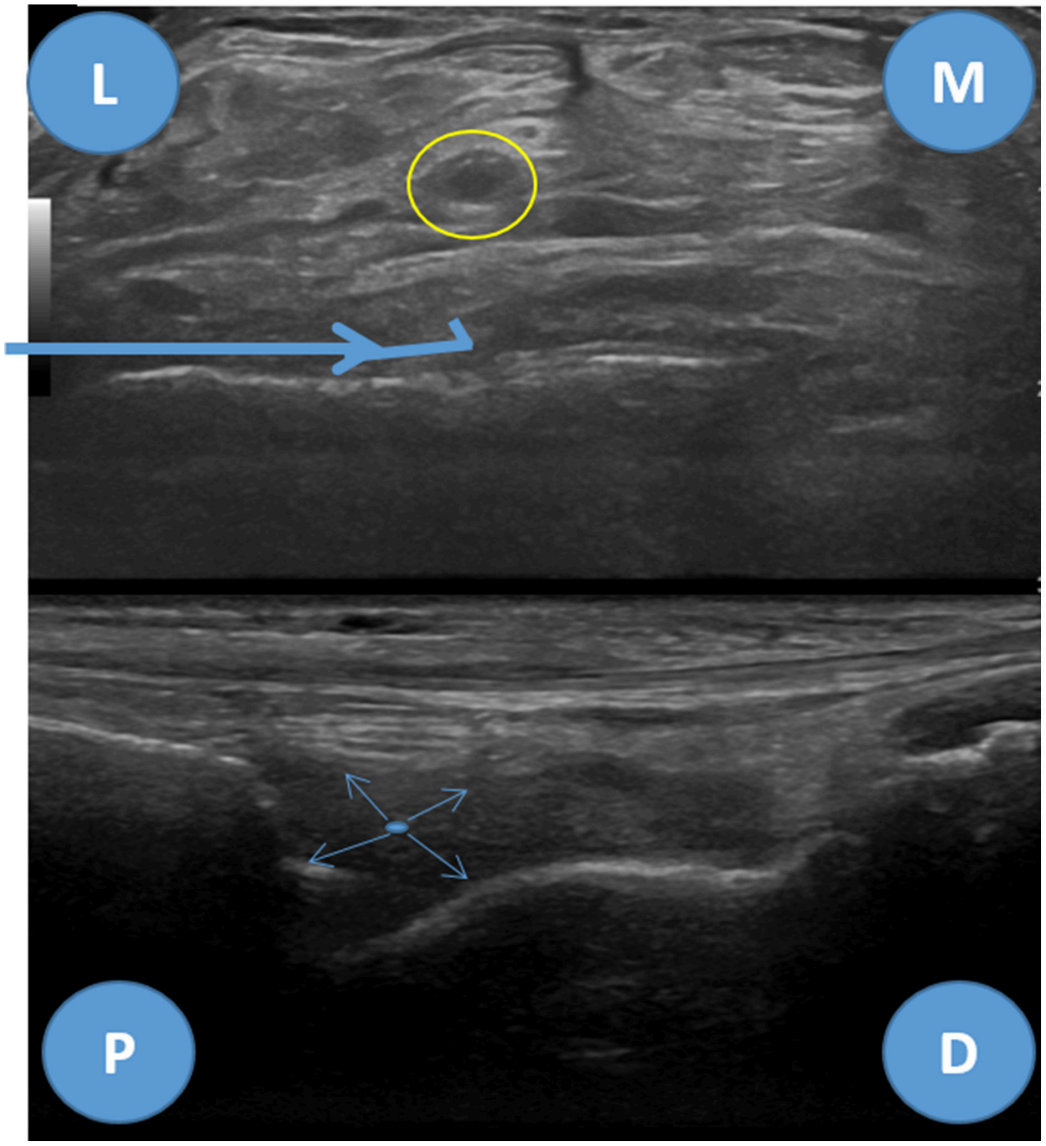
Anterolateral biopsy approach for the tibiotalar joint, ultrasound images. Upper image—transverse view of the tibitalar joint, L, lateral; M, medial. Yellow circle—tibialis posterior artery and deep peroneal nerve, Bold blue arrow—biopsy needle trajectory. Lower image—longitudinal view, P, proximal; D, distal. Thin blue arrows—needle throw directions.
